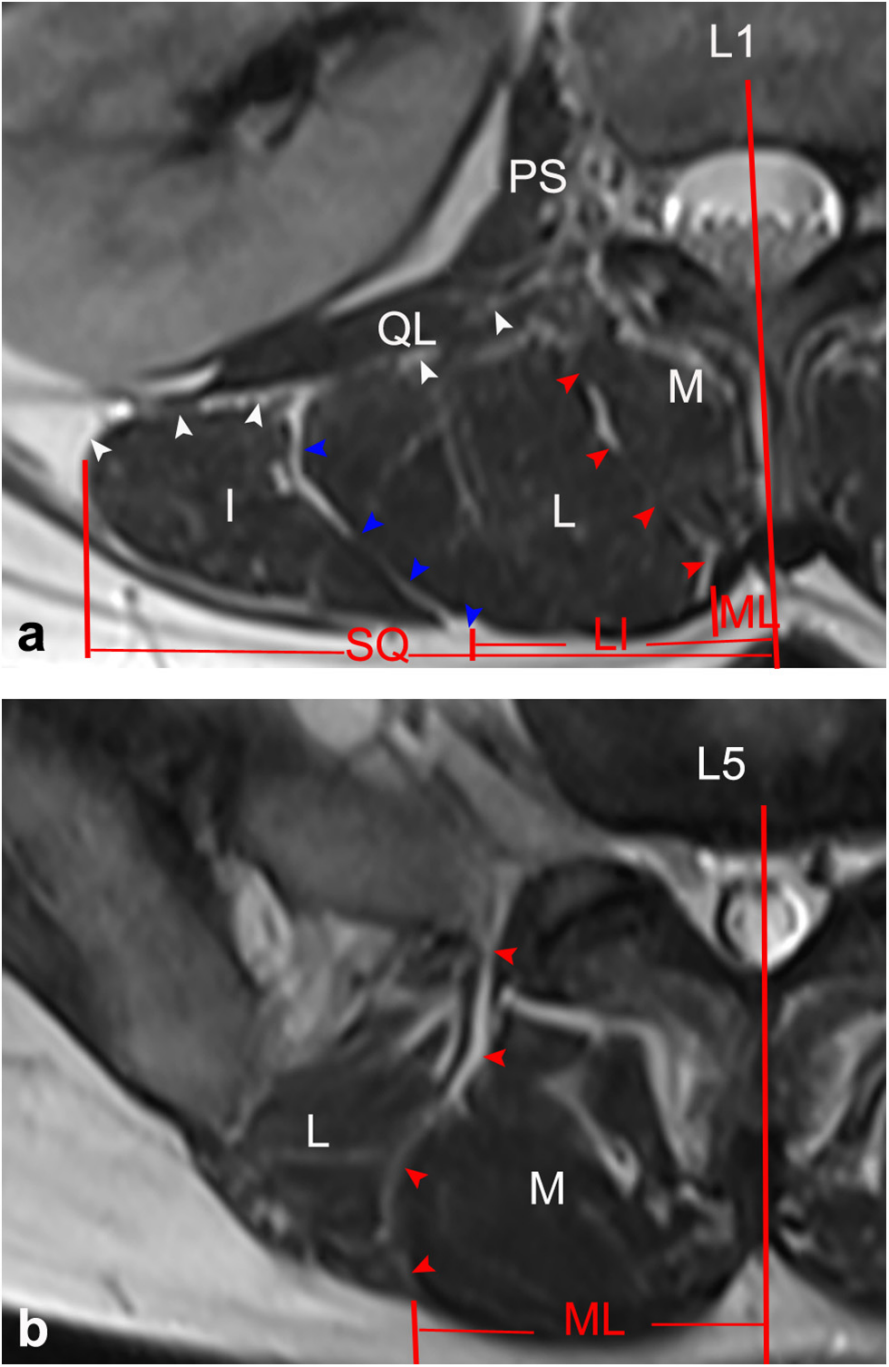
Horizontal views of the posterior lumbar spine in MRI images. (a) Through the upper level of the lumbar spine (L1), ML (red arrows), SQ (white arrows) and LI (blue arrows) were identified by lines of high signal intensity inserted into the paraspinal muscles. (b) Through the lower level of the lumbar spine (L5), only the ML was identified. *M*: multifidus; *L*: longissimus; *I*: iliocostalis; *IS*: iliac spine; *QL*: quadratus lumborum; *PS*: psoas major.
